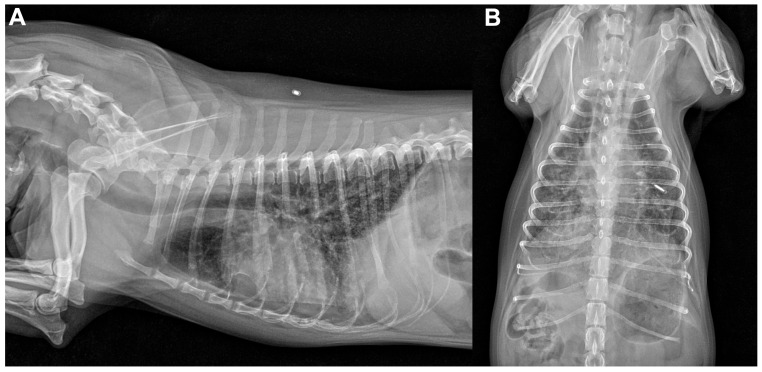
Right lateral (A) and ventrodorsal (B) thoracic radiographs obtained during one emergency visit show a marked, diffuse interstitial-to-alveolar pattern, most prominent in the caudal lung fields. Radiographic changes during other emergency visits were similar.
